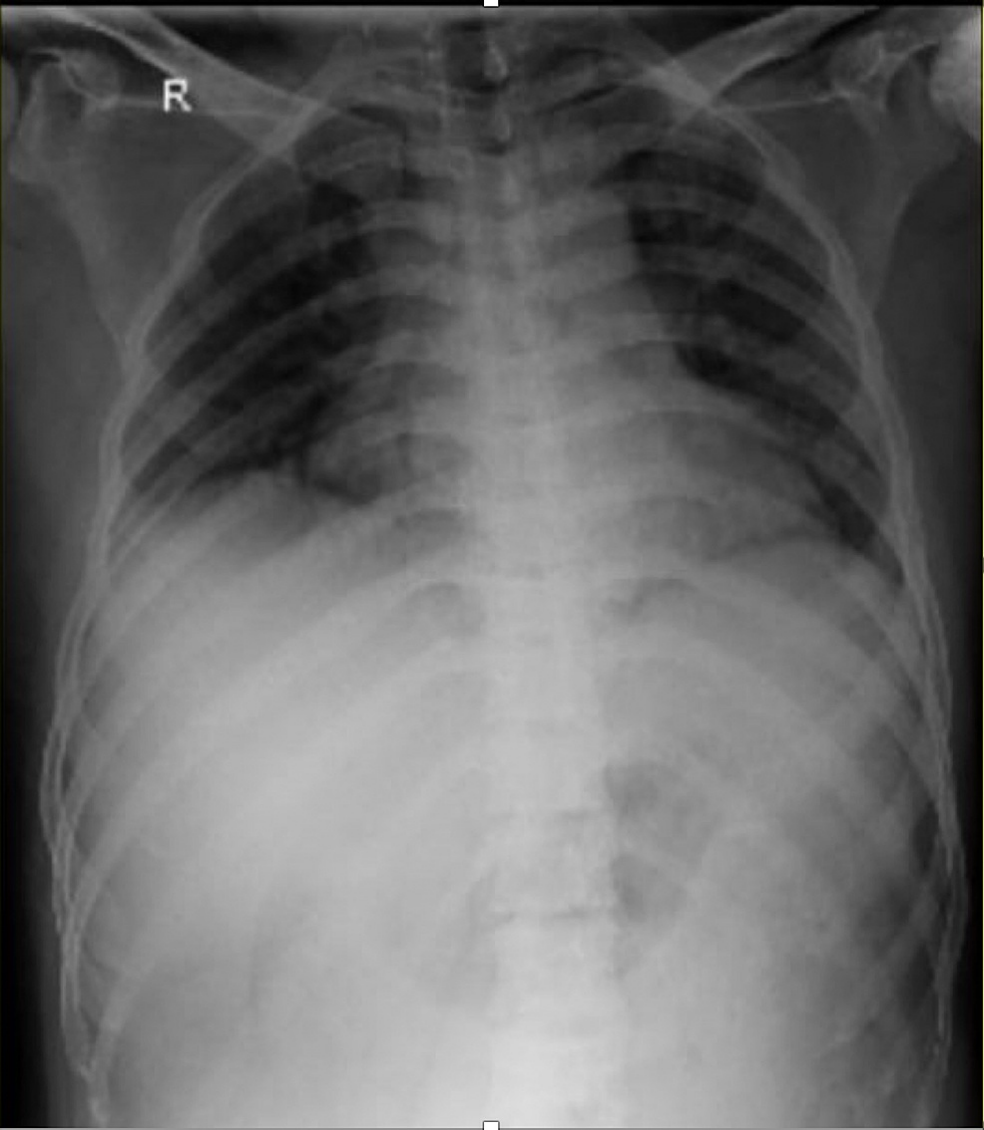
The image is showing a normal chest x-ray on admission
